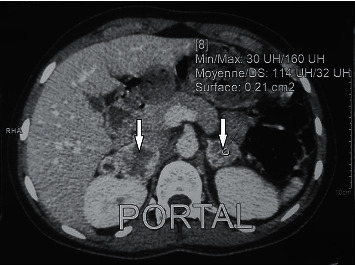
Abdominal computed tomography showing the two adrenal masses (two white arrows).
